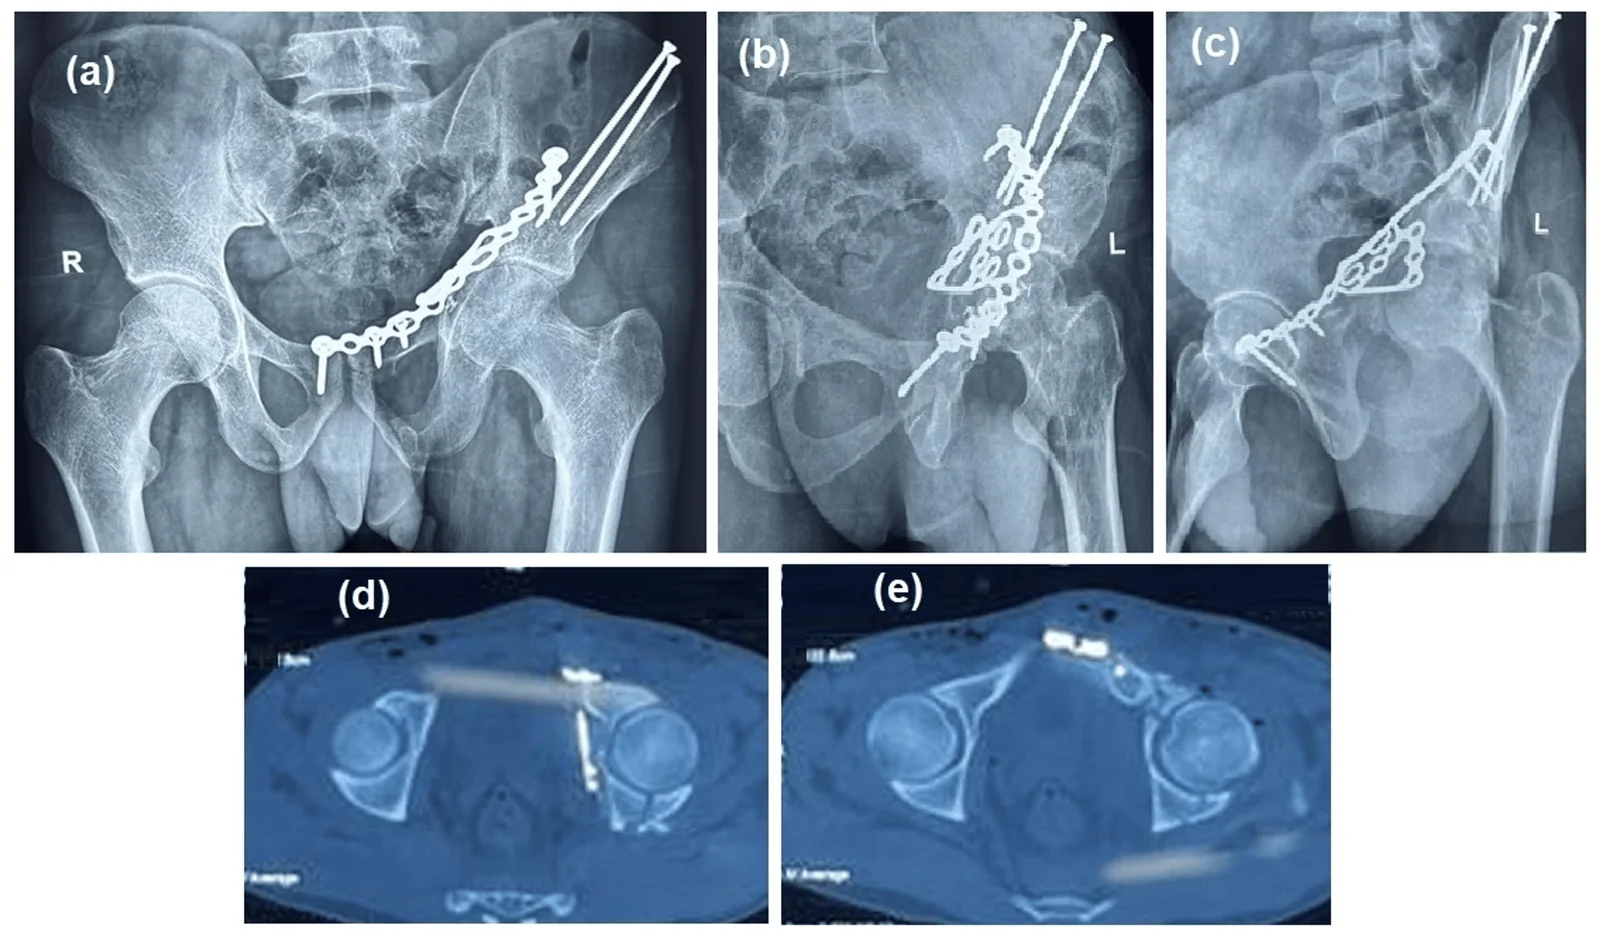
Case 1: Radiological follow-up. (a) Follow-up anteroposterior pelvic radiograph. (b) Follow-up iliac oblique radiograph. (c) Follow-up obturator oblique radiograph. (d) Follow-up axial CT image. (e) Follow-up axial CT image demonstrating maintained reduction and stable implant position.
